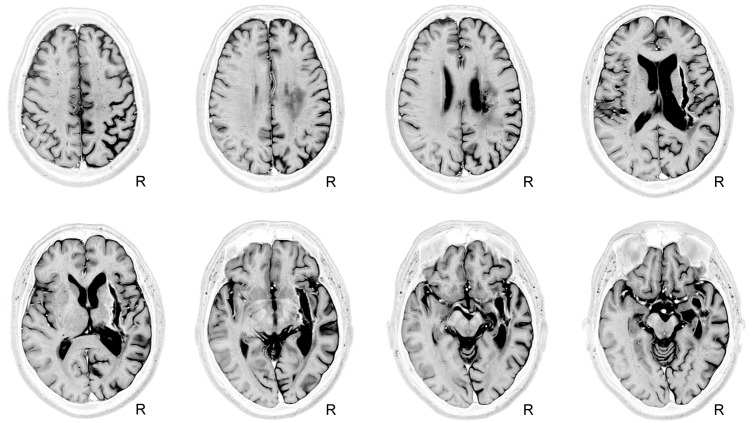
Depiction of an old right subcortical hemorrhage on a Short-TI Inversion Recovery (STIR) MRI sequence. Axial views of MRI-STIR images are shown in native space. The MRI shows an extensive lesion with a semilunar configuration involving the right striatum-capsular region extending into the surrounding white matter. Mild post-stroke right temporal-parietal cortical atrophy is evident. See text for further details. The neurological convention is used. R, right.
